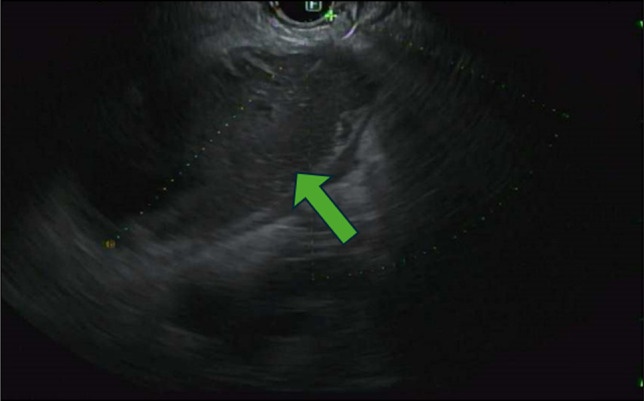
Endoscopic ultrasound from the rectum demonstrating a blind ending fluid-filled bowel loop adjacent to the rectal wall, consistent with a noncommunicating bowel segment (green arrow).
